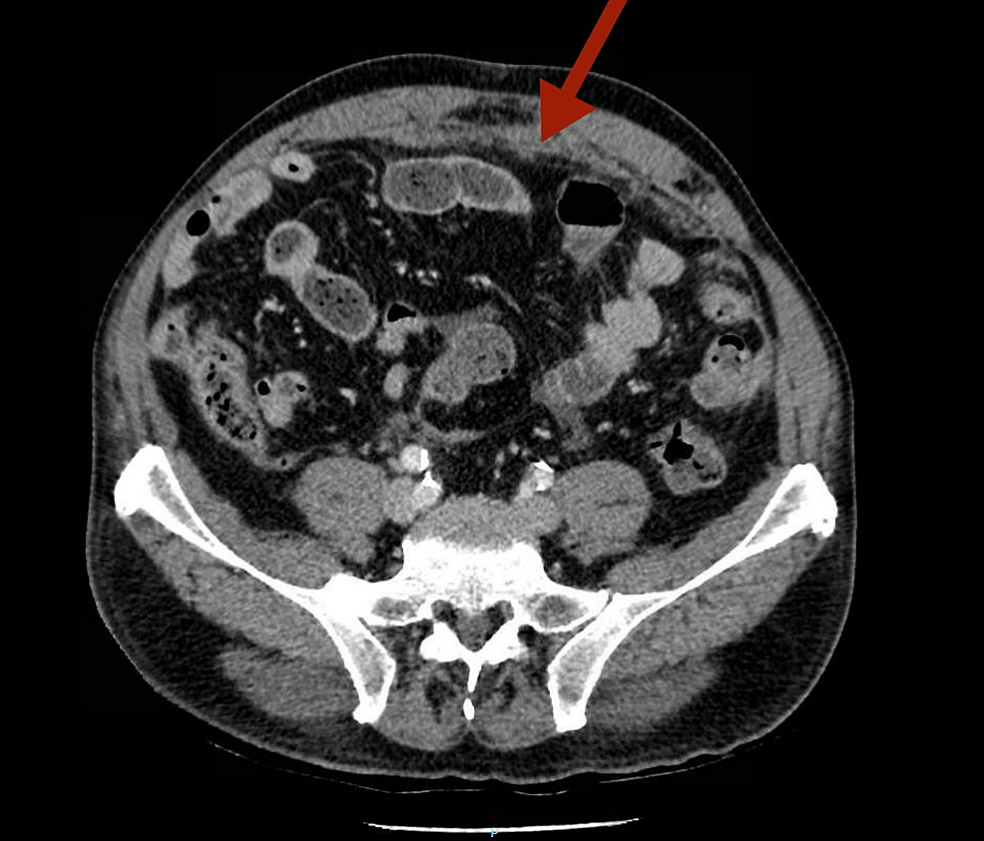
Abdominal CT scan: “omental cake” marked with a red arrow
